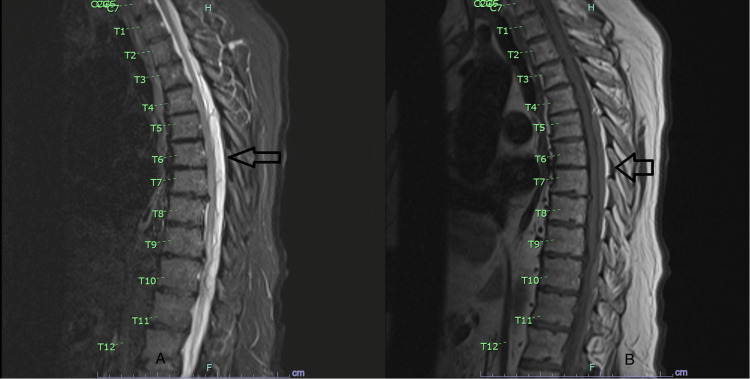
MRI short tau inversion recovery and T1 of the thoracic spine. (A) An MRI short tau inversion recovery sequence showing extensive posterior thoracic epidural hematoma. (B) An MRI T1 sequence showing similar findings with shortening and subtle enhancement (arrows).
